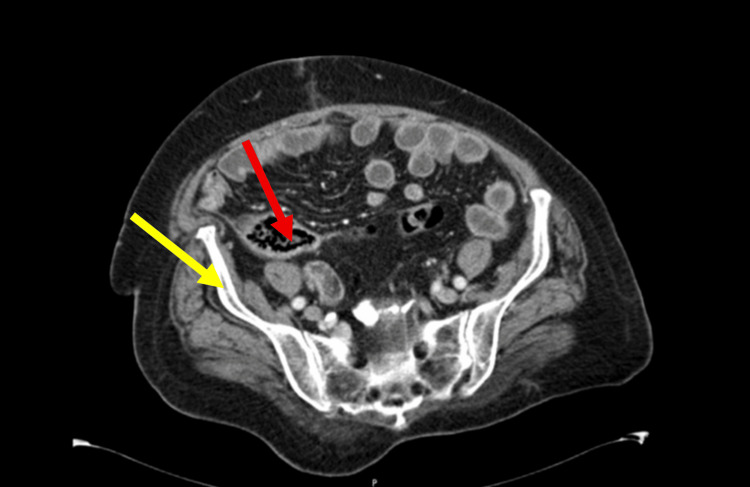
Postoperative CT abdomen showing the cecum low in position at the pelvic inlet and appears less distended. Axial pelvic CT slice obtained on follow-up demonstrating the cecum (red arrow) located deep within the pelvis. The structure is positioned immediately adjacent to the right pelvic bone (yellow arrow). No evidence of large bowel obstruction or colonic dilation is present in this scan.
